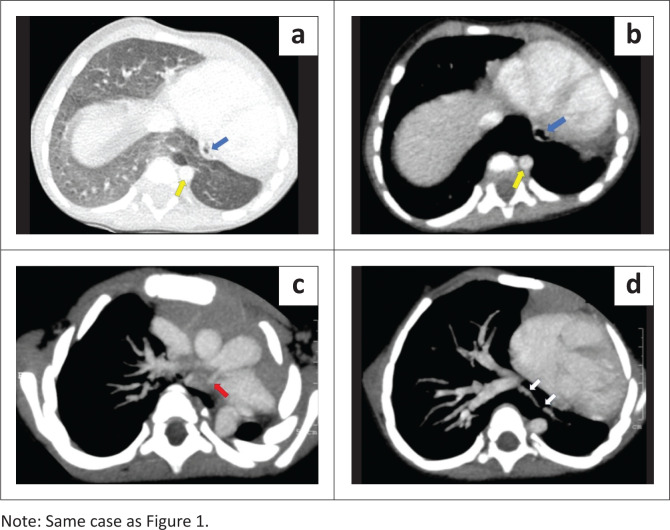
Contrast enhanced CT chest (a) lung window, (b) mediastinal window) images reveal that the fusion of the lung parenchyma lies posterior to the heart and the oesophagus (blue arrow) and anterior to the aorta (yellow arrow). Axial maximum intensity projection (MIP) images (c and d) demonstrate a hypoplastic left pulmonary artery at its origin (red arrow), and a single pulmonary vein (white arrows) draining the hypoplastic left lung, traversing through the isthmus of the true horseshoe lung and joining the right inferior pulmonary vein.
